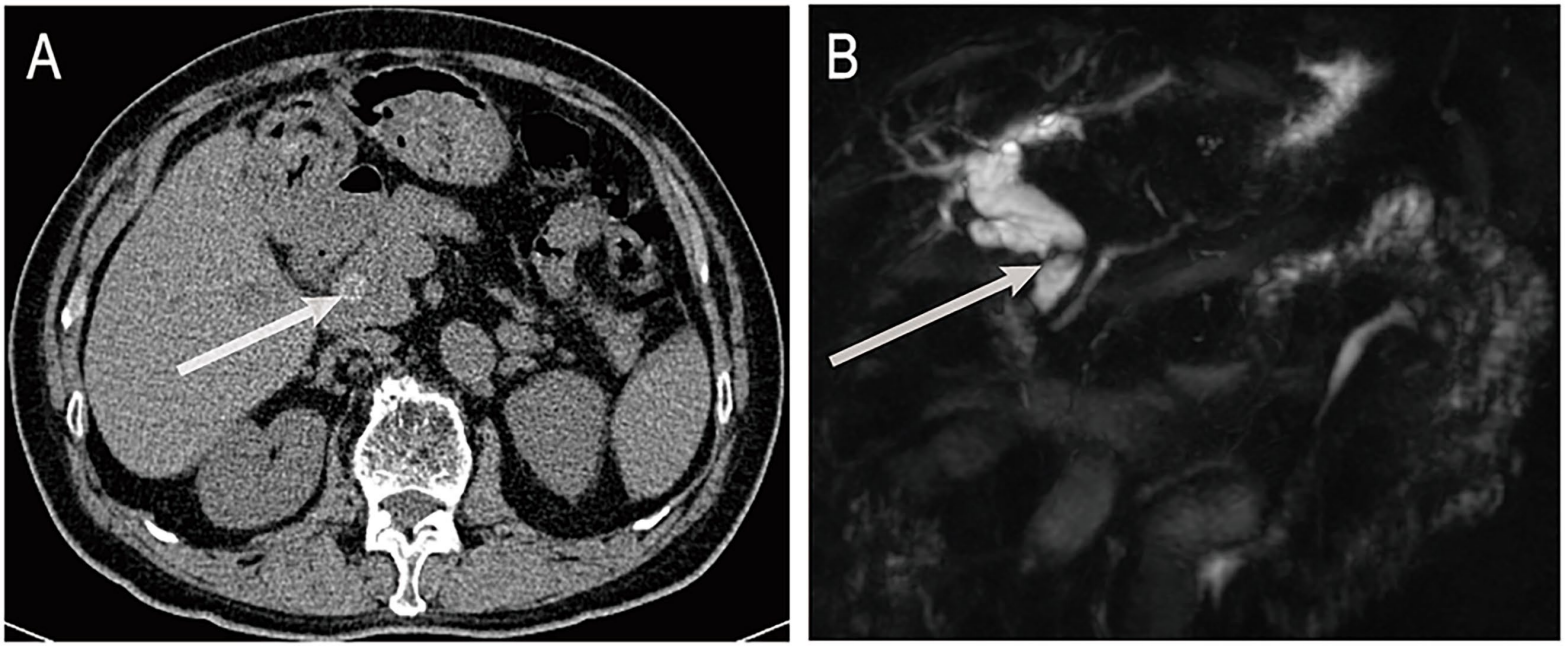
(A) Abdominal non-contrast CT shows a stone in the distal common bile duct. (B) MRCP reveals dilatation of intrahepatic and extrahepatic bile ducts with distal obstruction caused by a stone. ERCP, endoscopic retrograde cholangiopancreatography; CT, computed tomography; MRCP, magnetic resonance cholangiopancreatography. The white arrows indicate bile duct stones.
